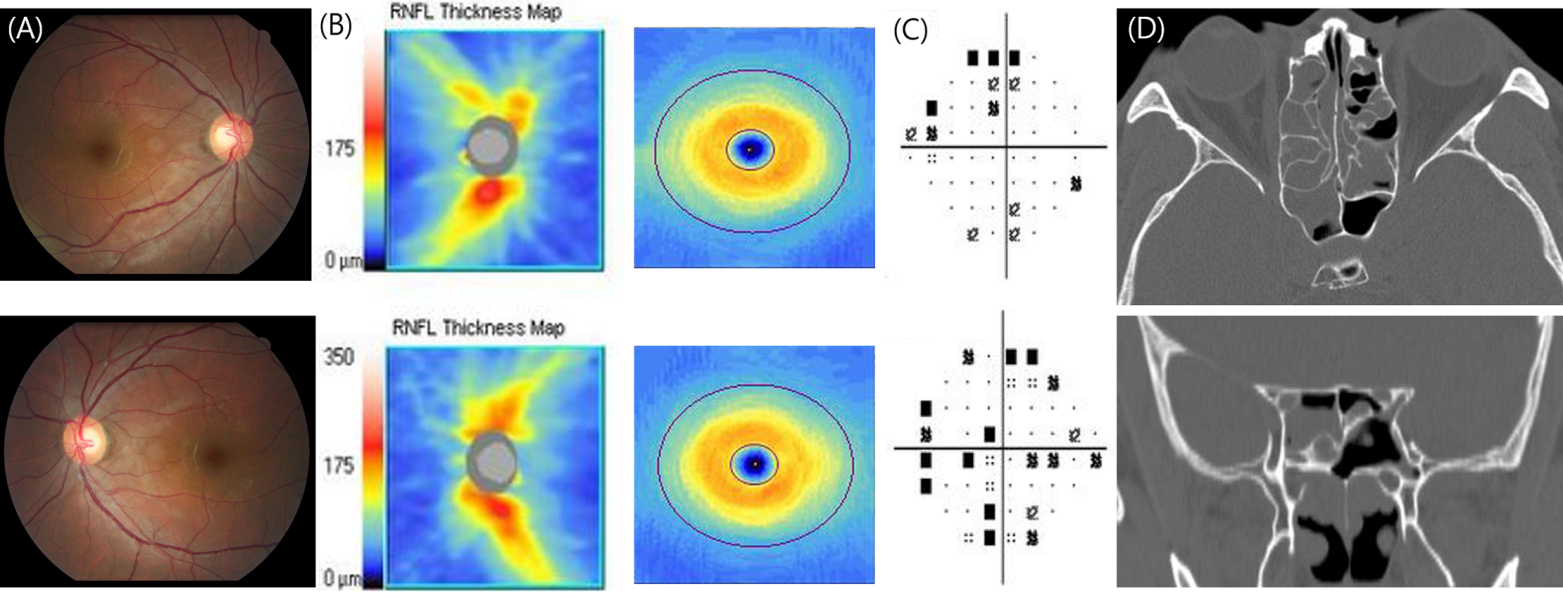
Case. (A) The optic discs appear normal on fundus photography (upper, right eye; lower, left eye). (B) On the OCT RNFL map, there is subtle thinning of the superior aspect of the retinal nerve fiber layer in the right eye (upper, right eye; lower, left eye). OCT GCIPL map shows normal finding. (C) Reliable automated perimetry demonstrates generalized decreased sensitivity in both eyes (upper, right eye; lower, left eye) (mean deviation -4.62 dB, pattern standard deviation 5.40 dB in his right eye; mean deviation -7.85 dB, pattern standard deviation 5.68 dB in his left eye). (D) The ostiomeatal CT image shows sinusitis of the posterior ethmoid and sphenoid sinuses. In addition, the optic nerve protrudes into a well pneumatized posterior ethmoid sinus (Onodi cell) with some bony dehiscence.
